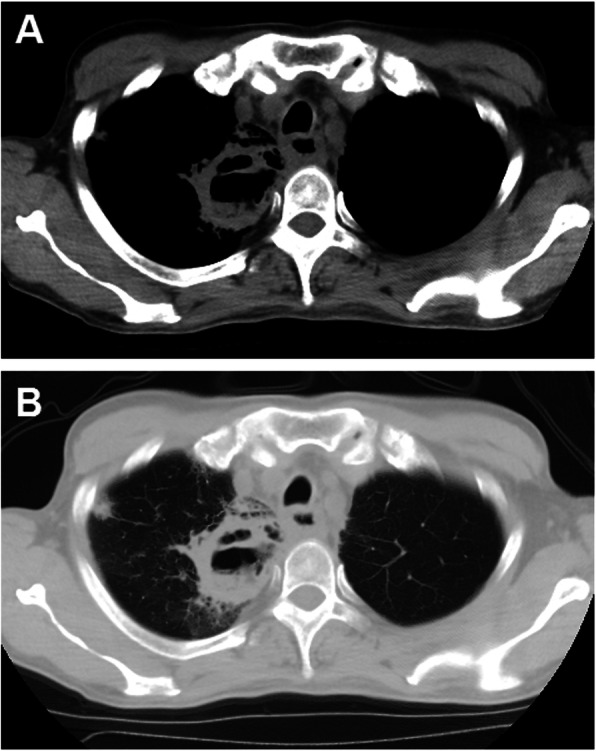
Chest CT scans for 66-year-old man with esophageal fistula and stricture. Chest CT scan in the mediastinal (a) and lung windows (b) show mediastinal abscess without obvious pleural effusion before procedure
